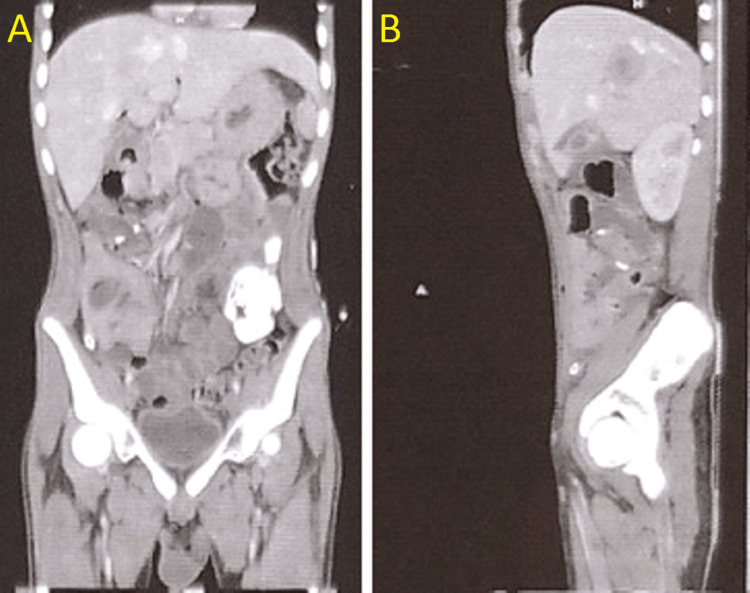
Coronal section of the contrast-enhanced computed tomography scan of abdomen pelvis showing multiple liver lesions Panel A shows a lesion in segment V of the liver. Panel B shows a lesion in the liver with central cystic and peripheral solid components.
